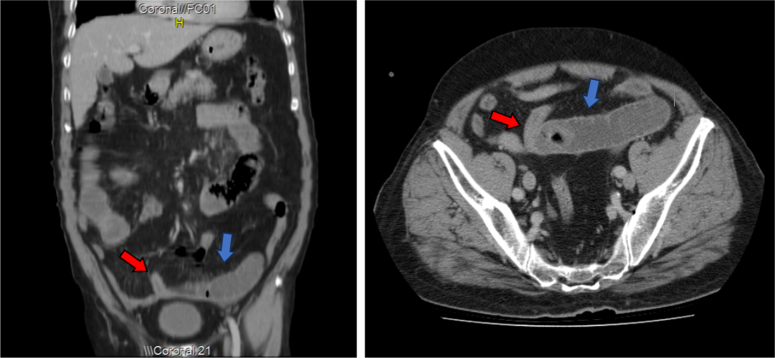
The object obstructing the bowels on the patient’s computed tomography scan of his pelvis and abdomen. (Red arrows) with the transitional dilated zone in intestinal loops (blue arrows).
